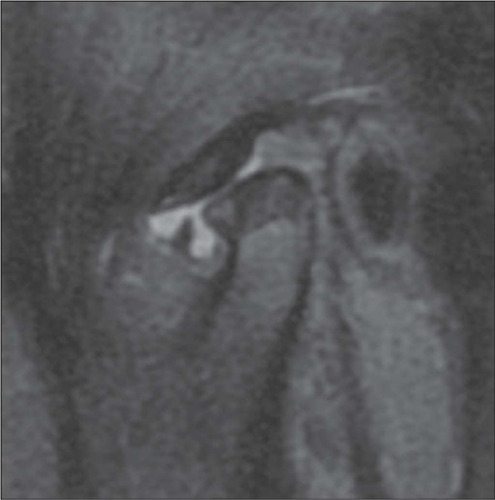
Joint effusion. Sagittal T2-weighted image with fat saturation, showing joint
